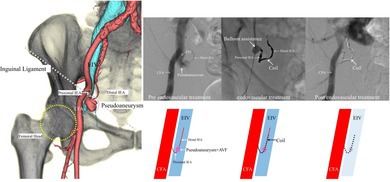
Pulmonary vein (PV) isolation with pulsed field ablation (PFA) for atrial fibrillation (AF) is an emerging therapy with myocardial selectivity that provides efficacy comparable to radiofrequency ablation while reducing complications [1]. However, vascular complications may still occur despite ultrasound‐ and fluoroscopy‐guided puncture, because multiple punctures and large‐bore sheaths are often required. We report an asymptomatic patient who developed both a pseudoaneurysm and an arteriovenous fistula (AVF) at a single femoral puncture site.
